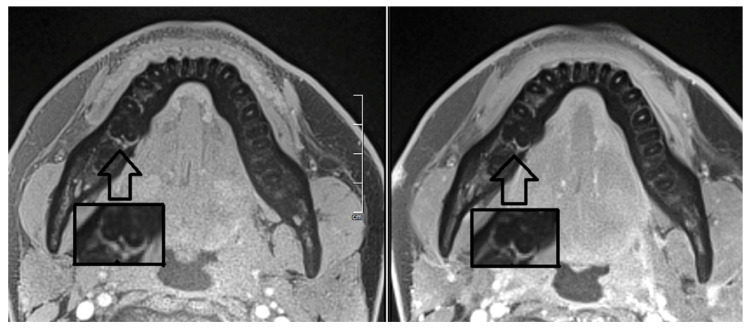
T1-weighted MRI on our 3T scanner, left without contrast and right with contrast. There is no significant T1 signal enhancement in the pulp in both native and post-contrast imaging. Note: Healthy teeth show clear and measurable contrast enhancement after magnification. The black arrow shows the region of interest with the image below magnified.
